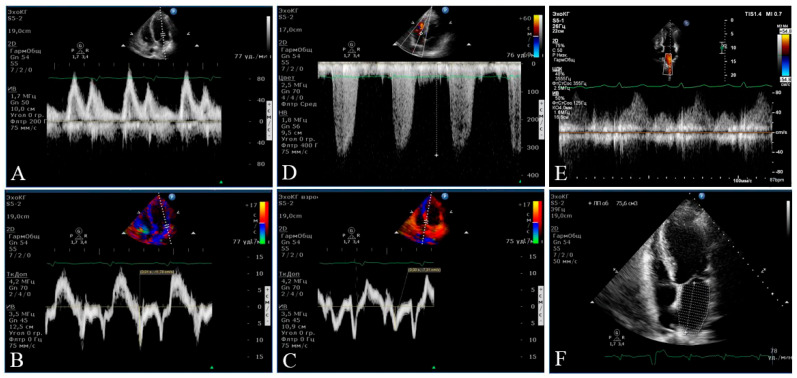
An episode of echocardiography in Participant B (female, 59 years old) with the 2nd stage of left ventricular diastolic dysfunction. In the Doppler mode (A), transmitral blood flow is shown with an assessment of Peaks E and A. In the tissue (B,C), the Doppler mode and the fibrous ring of the mitral valve movement are shown in the left ventricle early filling phase (Peaks e′ lateral and medial). (D) demonstrates the velocity of tricuspid regurgitation. (E) reflects the measurement of the flow in the pulmonary veins. The four-chamber position for measuring the volume of the left atrium is shown in (F).
